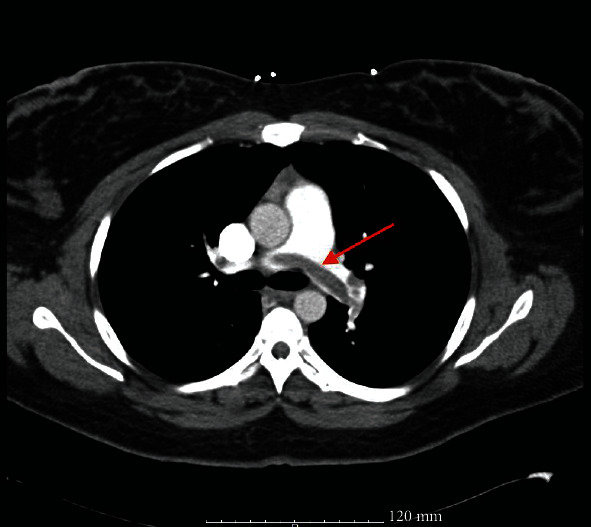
Computed tomography of the chest, transverse view, demonstrated a large saddle embolus as indicated by the red arrow.
